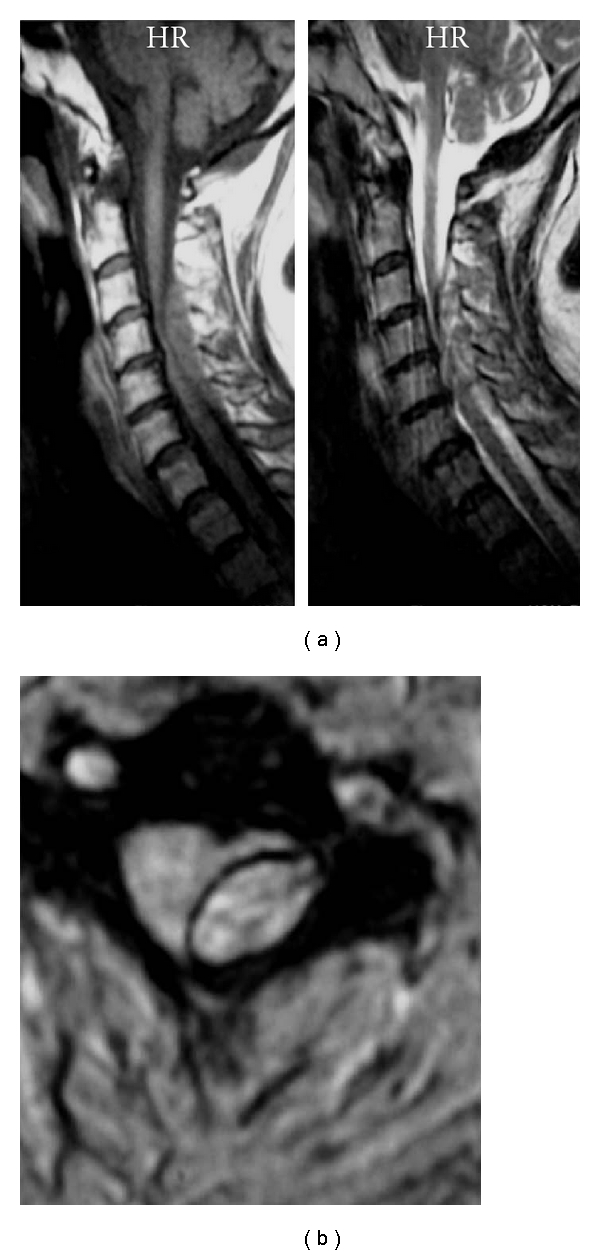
Preoperative sagittal (a) and axial (b) MR images showing a left posterolateral epidural hematoma at the C3-C6 level with spinal cord compression.
